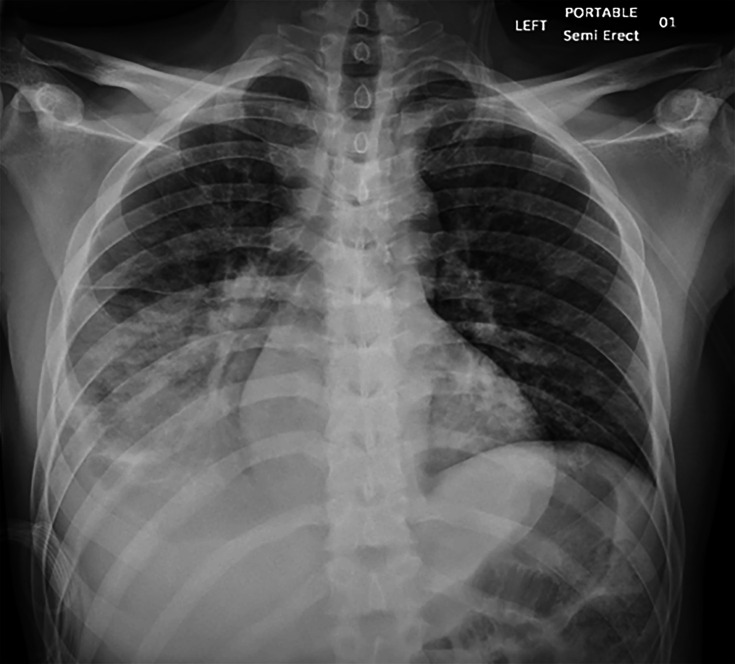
Chest X-ray from admission demonstrating right lower zone radiopacity with air bronchograms.
